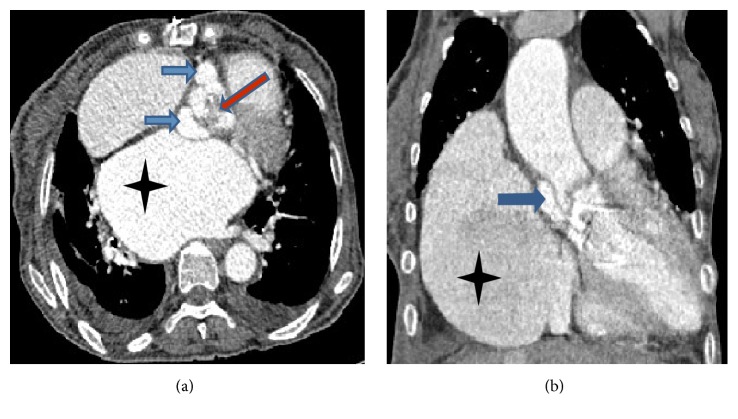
CT displays (a) on axial plane an irregular, deformed aortic valve, in which there is hypodense material (red arrow), two pseudoaneurysms (blue arrows), and dilated left atrium (black star). (b) Coronal reconstruction showing a pseudoaneurysm at the base of the ascending aorta (blue arrow) and dilated left atrium (black star).
